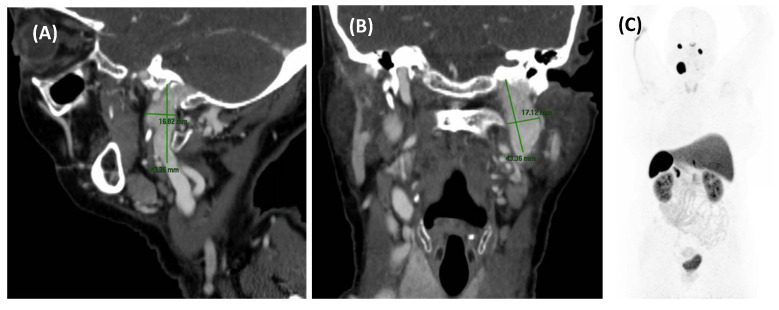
A CT scan (sagittal section, image (A); coronal section, image (B)) in a 76-year-old woman with somatosound in the left ear for 3 years. It reveals an area of intense contrast uptake, with a larger craniocaudal diameter, located at the left basal cranial level which, due to its location, is suggestive of a jugular paraganglioma. The SPECT-CT scan with 111In-pentetreotide (image (C)) did not show other foci of tracer uptake, suggesting the absence of a neuroendocrine tumor with positive somatostatin receptor expression elsewhere in the body. This patient was treated with radiotherapy with a good response.
